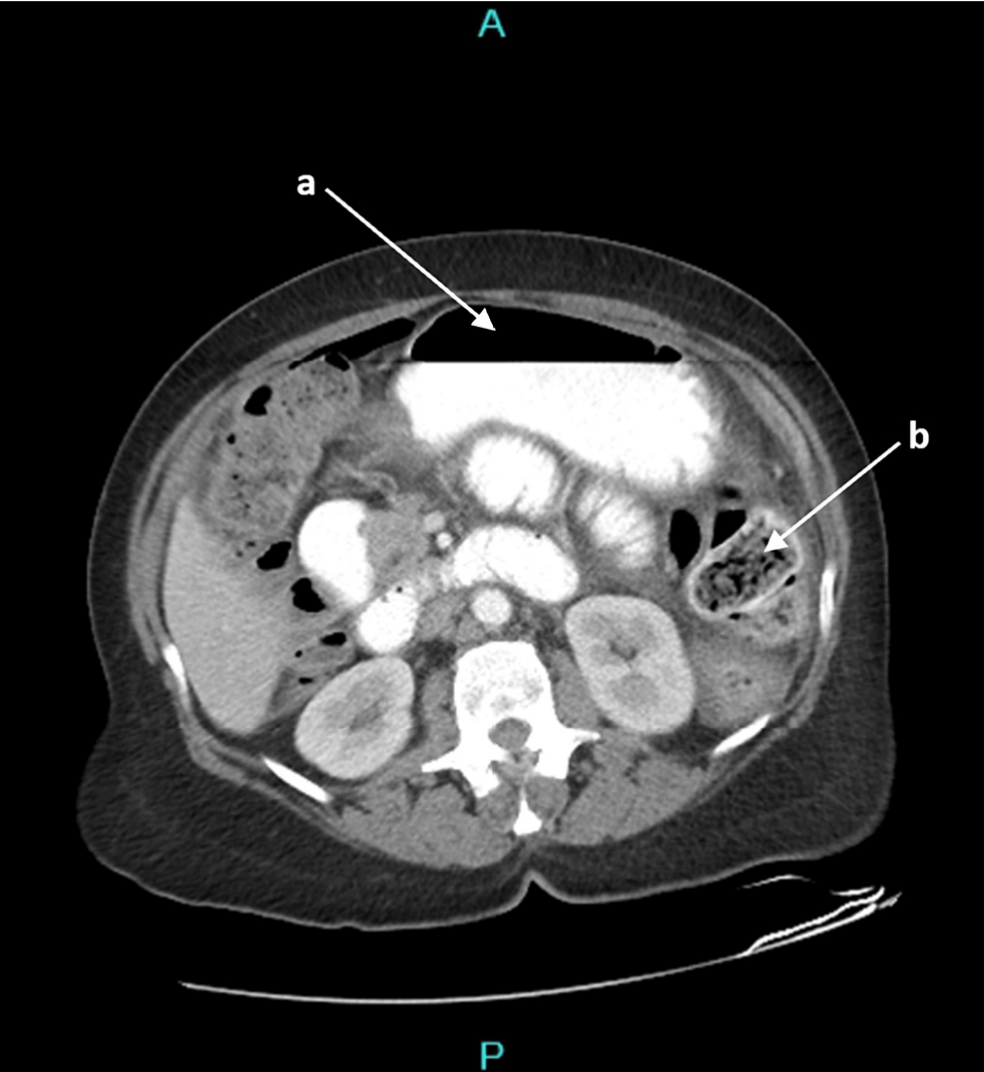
CT abdomen with oral contrast The image is demonstrating significantly distended loops of bowel with air-fluid level and stool burden. Arrow a demonstrates air-fluid level and bowel distention. Arrow b points to stool burden in one loop of bowel
